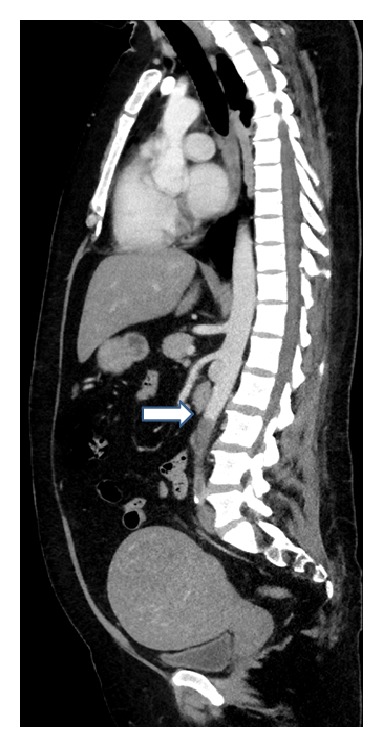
Abdominal computed tomography sagittal view demonstrates embolus (arrow) in the distal aorta to the level of the third lumbar vertebra.
